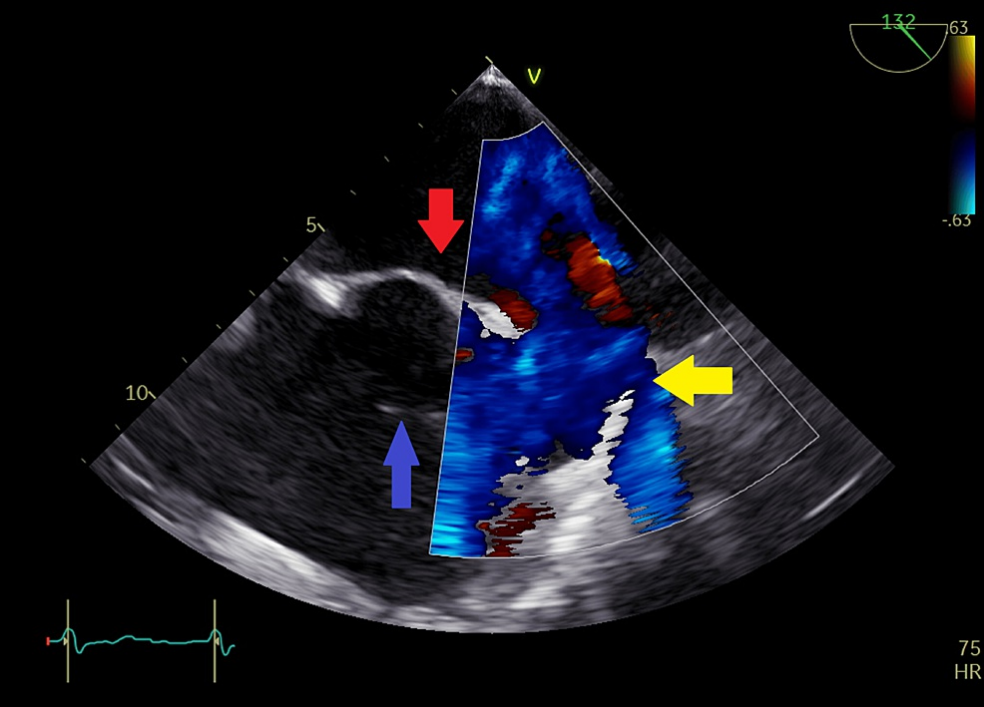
Transesophageal echocardiography at the level of left atrium and right atrium with color flow coding at the sinus venosus site showing left to right shunt across the sinus venosus atrial septal defect. Yellow arrow shows color-coded left-to-right shunt; blue arrow in right atrium shows part of the cor triatriatum dexter membrane; red arrow in left atrium shows intact osteum secundum septum
